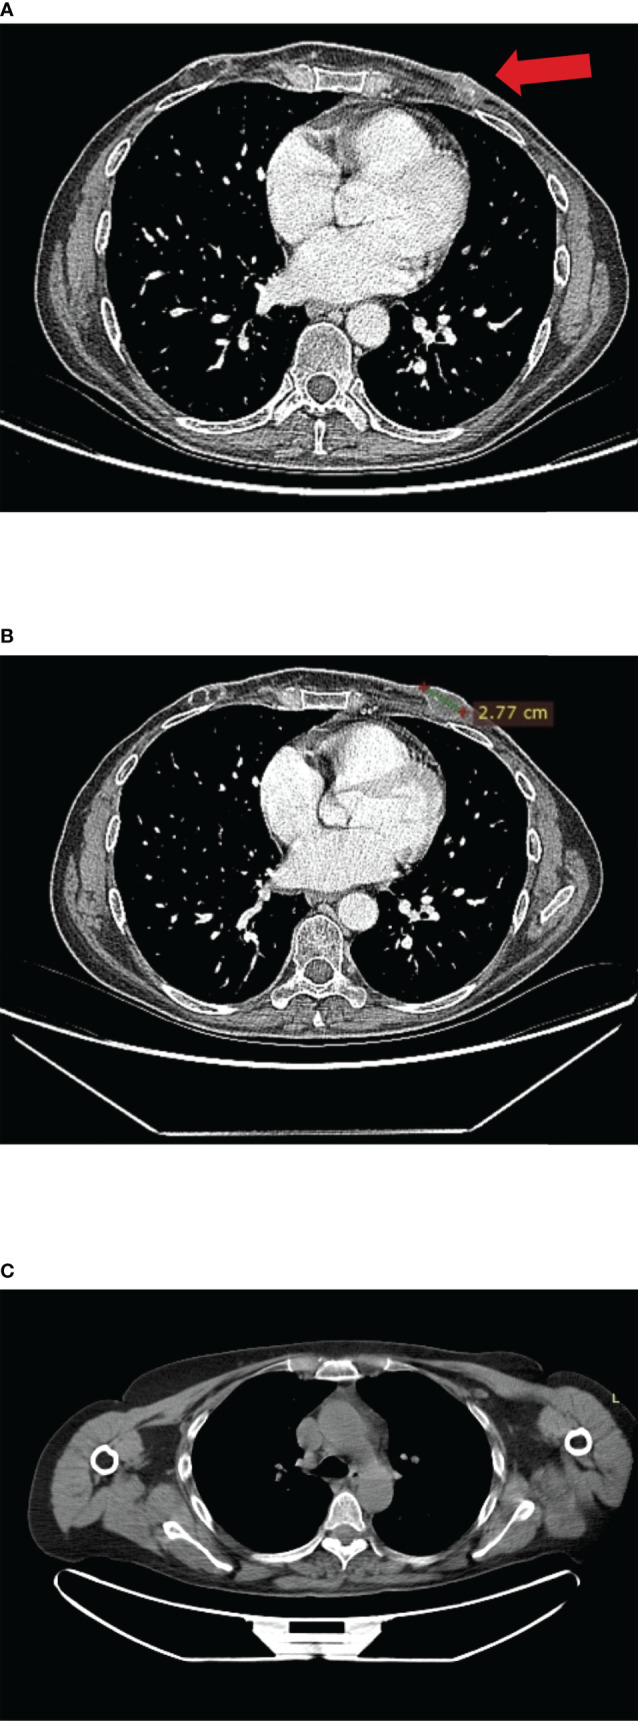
(A) CT scan images of left chest wall disease progression at enrolment in the IMpassion131 trial. (B) CT scan images at enrolment in CLAG525B2101 trials. (C) Complete response after 6 months of treatment with olaparib; no contrast enhancement at PET-CT scan.
